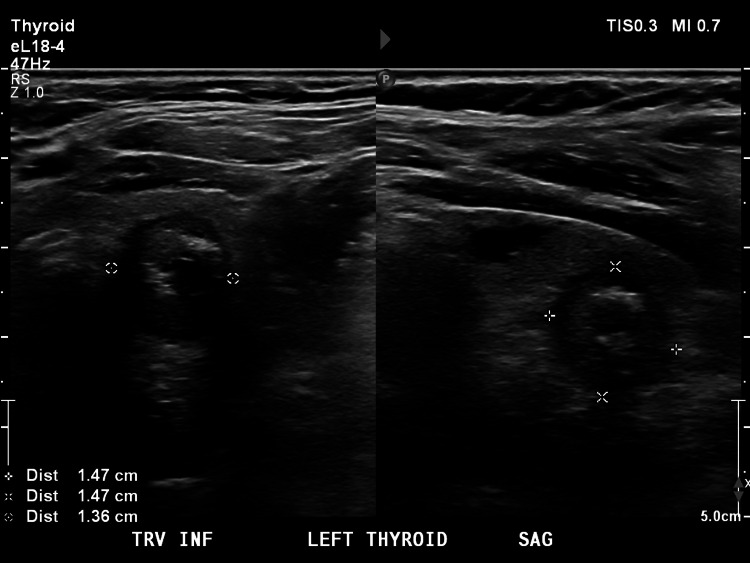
Ultrasound of thyroid gland demonstrating two nodules of the left inferior thyroid pole. ACR TI-RADS is a systematic approach to identifying if a thyroid nodule is suspicious for malignancy. It accounts for composition, echogenicity, shape, margin, and echogenic foci to determine the suspicion. The 1.47cm x 1.47cm x 1.36cm nodule labeled in the figure is considered highly suspicious (ACR > 7) according to the TI-RADS criteria due to the solid composition, hypoechoic nature, and echogenic foci. TRV: transverse; INF: inferior; SAG: sagittal; ACR: American College of Radiology; TI-RADs: Thyroid Imaging Reporting and Data System
